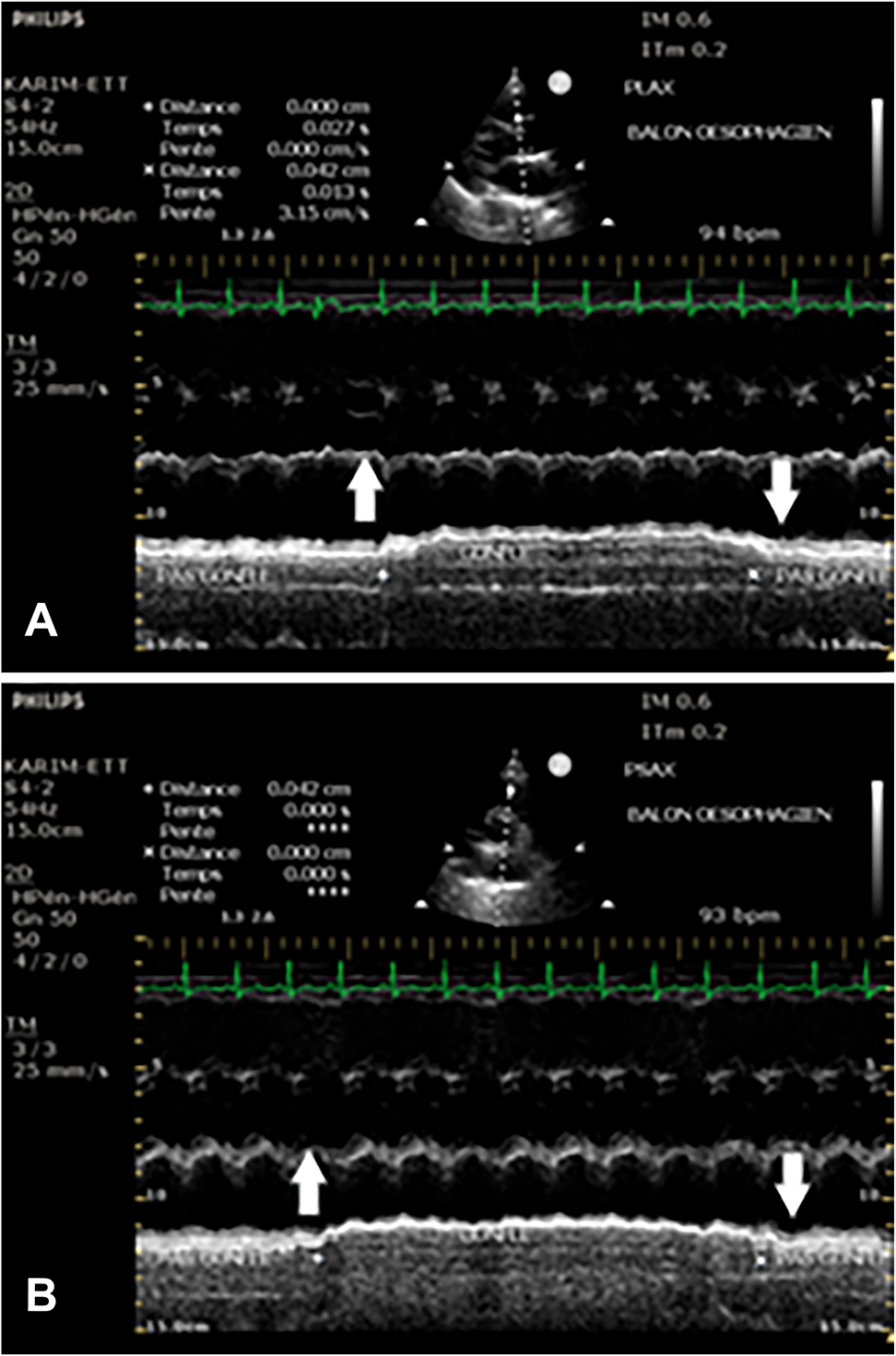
Indirect visualization of esophageal balloon by M-Mode in parasternal windows: A PLAX with M-Mode aiming the posterior wall of the left atrium. After the injection of 4 cc of air in the esophageal balloon we note an anterior displacement of the posterior wall of the atrium (upwards arrow) marking the presence of the esophageal balloon behind the atrium. After deflating the balloon we note the line of the posterior wall of atrium returning to the original position (downwards arrow); B The same method to visualize the balloon is used in PSAX showing the same reversible anterior displacement of the posterior wall of the atrium
